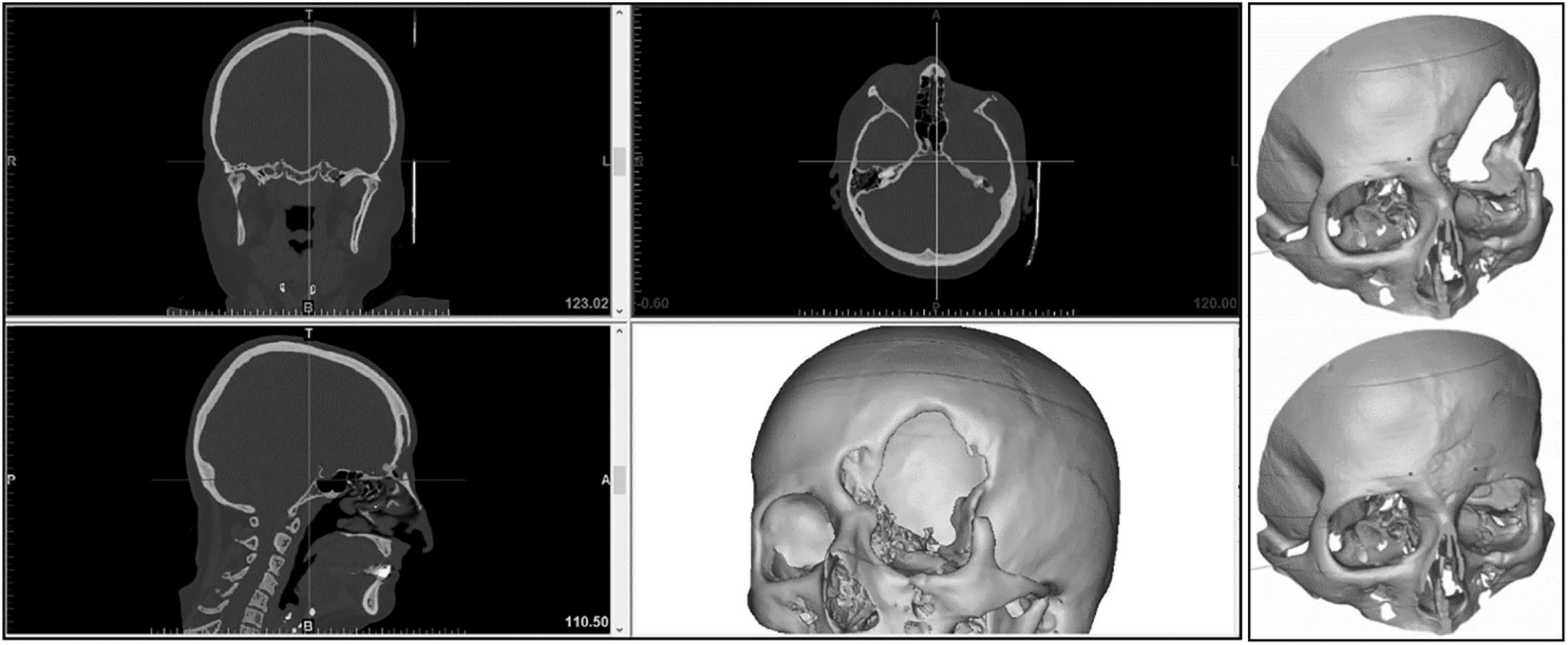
3D reconstruction based on CT scans and the design process using the mirroring technique. Image was created by the authors with Materialise Mimics 21.0 software (URL: https://www.materialise.com/en/healthcare/mimics-innovation-suite).
